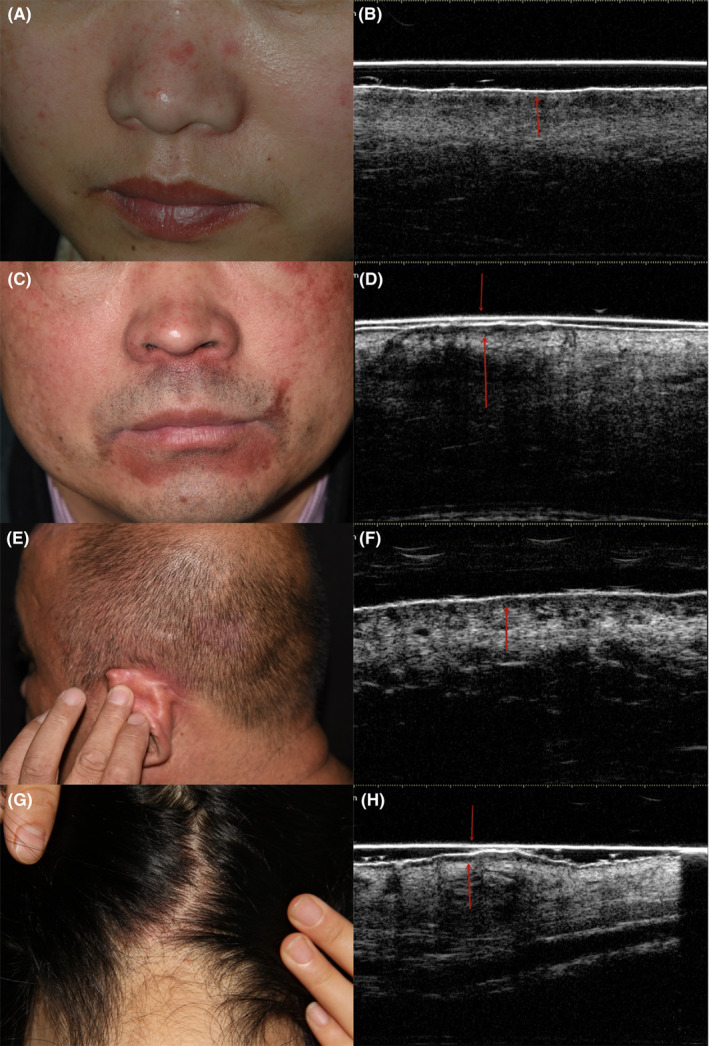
Clinical and ultrasonographic images of SD patient. 2A, 2C, 2E, 2G Macroscopic images. 2B, 2D, 2F, 2H Corresponding ultrasonographic images. 2D, 2H echo enhancement and unevenness of epidermis (
